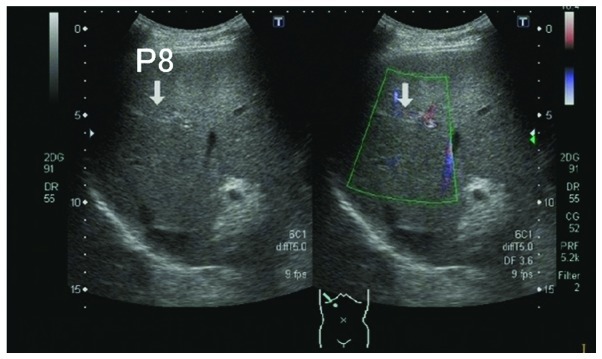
Abdominal ultrasonography (US) findings on hospital day 22 show the remaining thrombus in the P8 branch of the portal vein (arrow).
